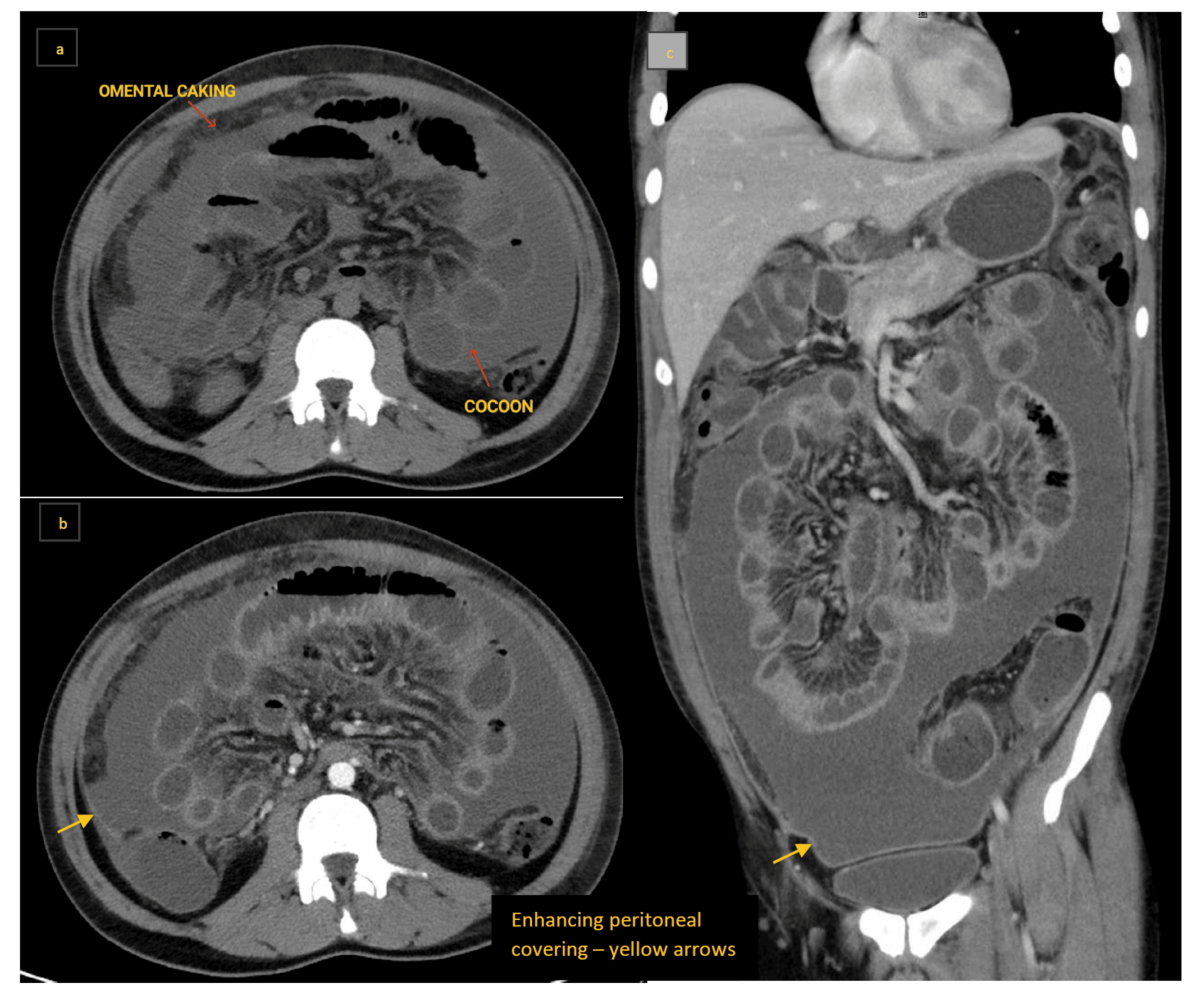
a: Axial non-contrast CT showing encapsulated ascites forming an "abdominal cocoon" with diffuse, smooth omental nodules suggestive of peritoneal TB. b: Axial contrast-enhanced CT with peritoneal enhancement. c: No evidence of proximal bowel dilatation, obstruction, or peritoneal/bowel wall calcifications. CT: computed tomography, TB: tuberculosis
